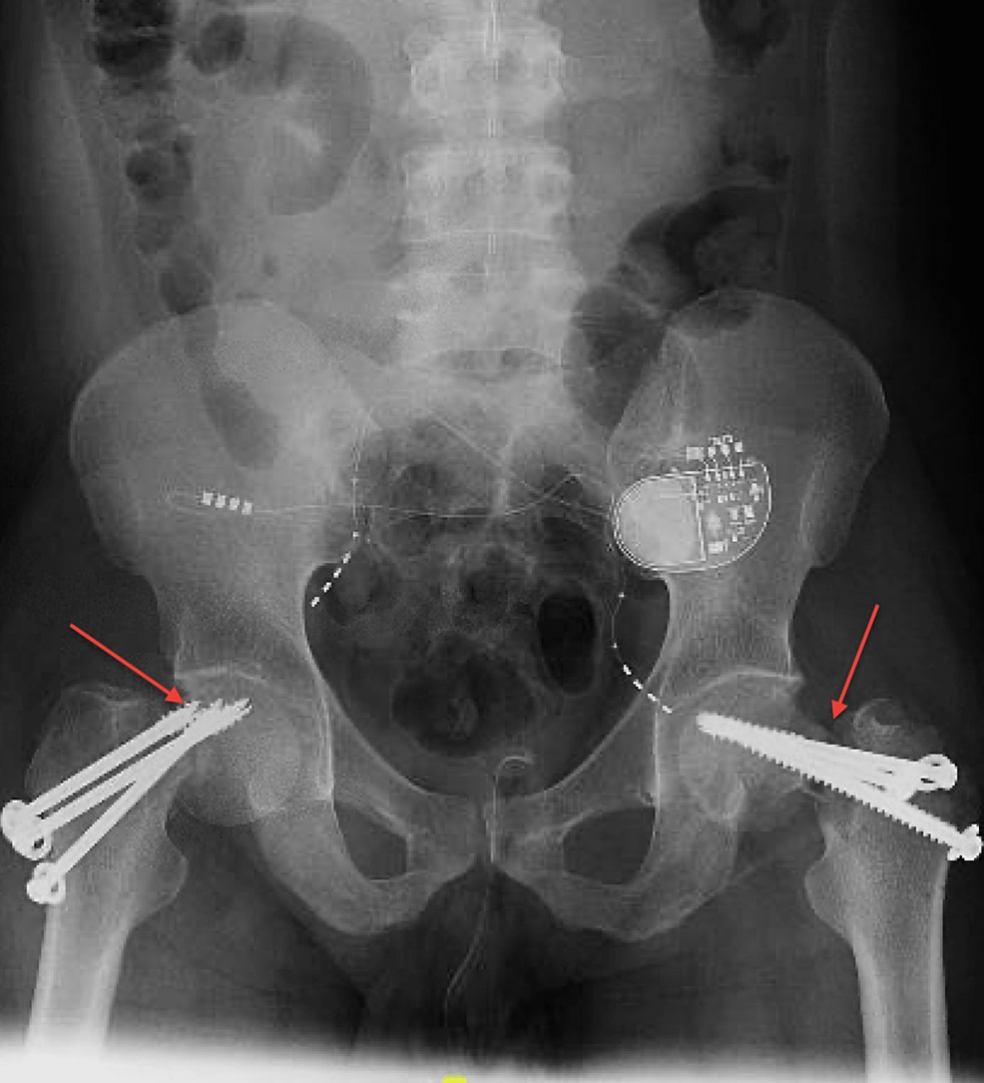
Postoperative x-ray after close reduction and cannulated screws
